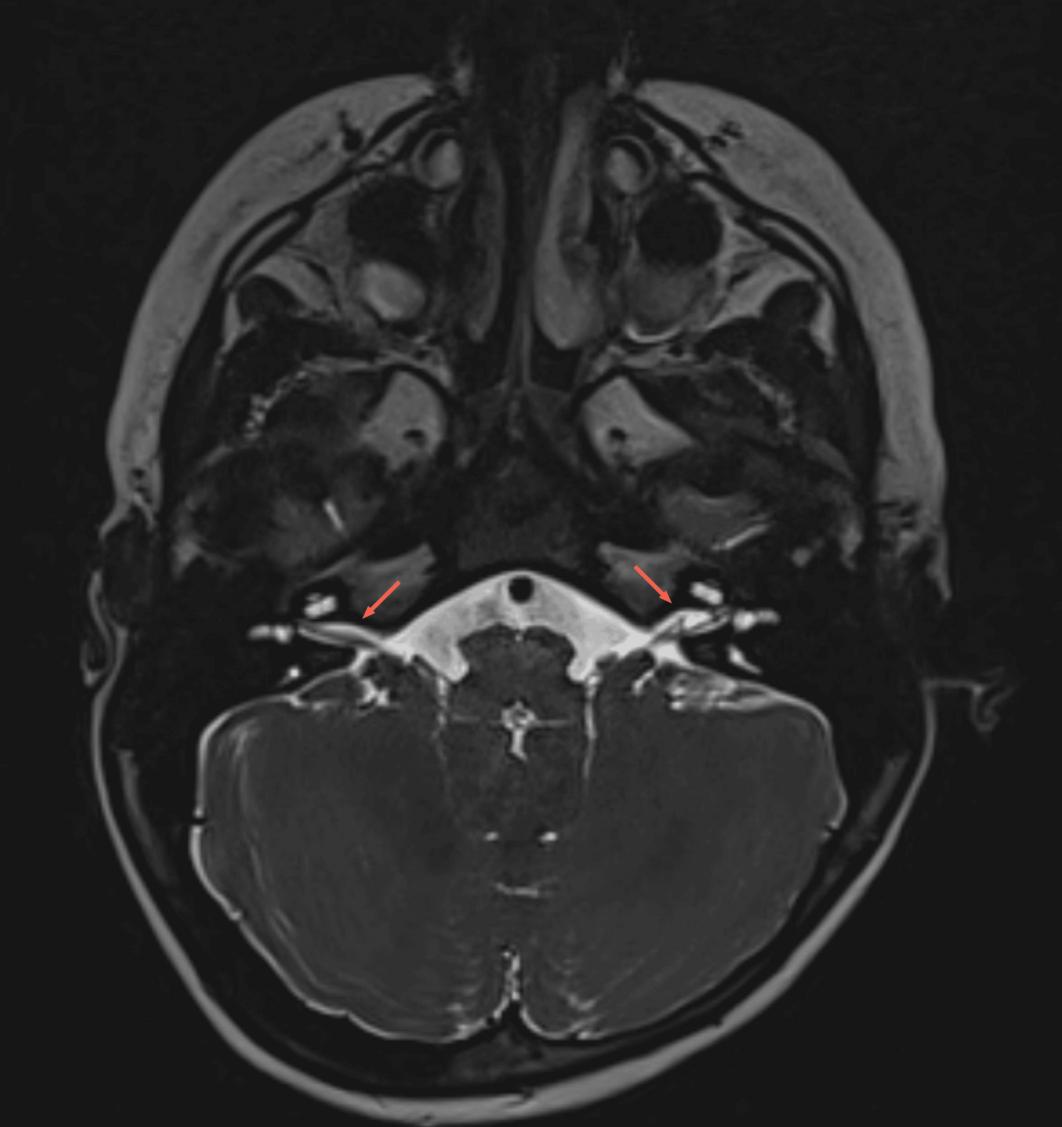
Magnetic resonance image with the bilateral internal auditory canals appearing normal (arrows)
